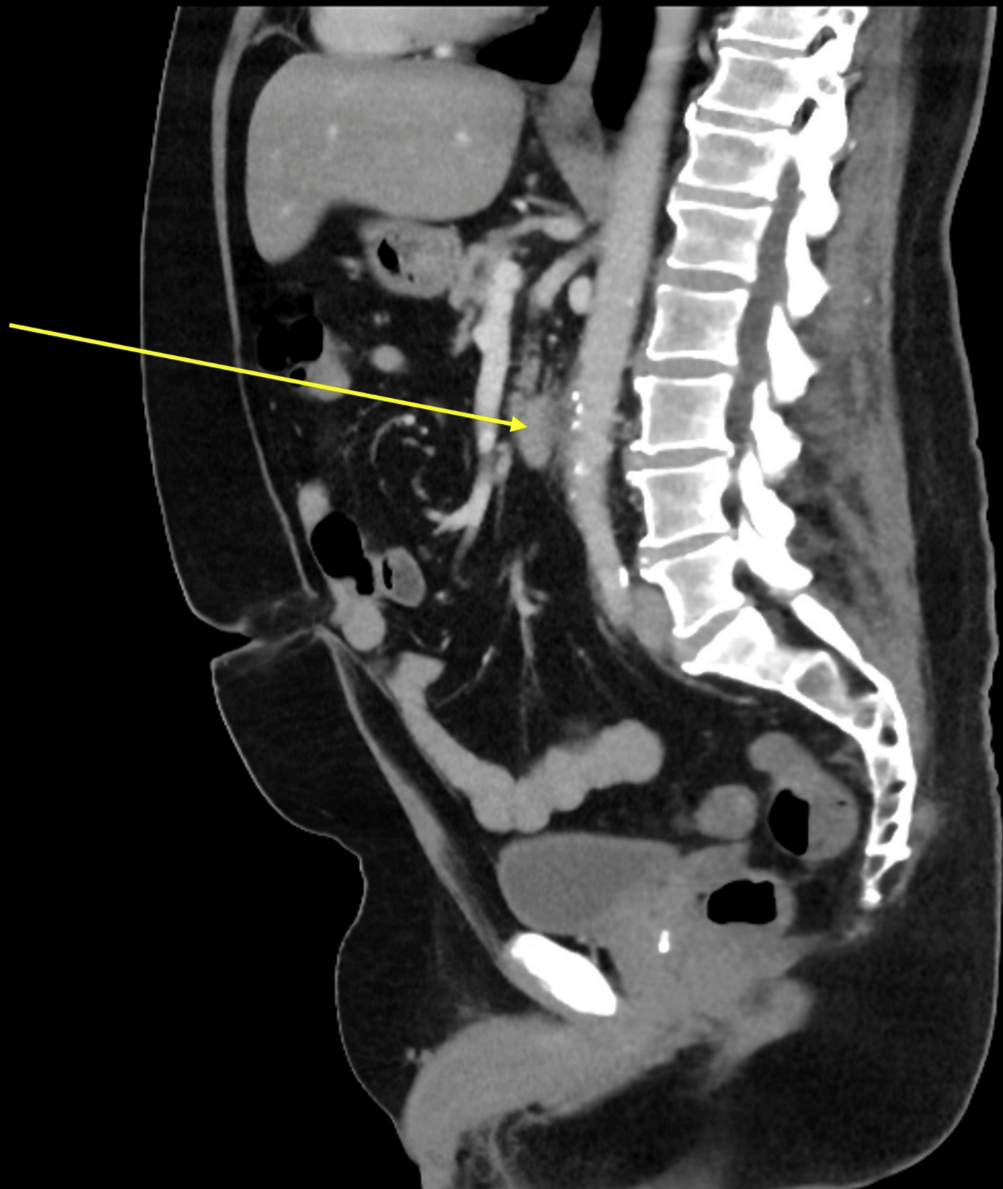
Sagittal contrast-enhanced CT of the abdomen and pelvis showing fusiform bulging of the mid-abdominal aorta with surrounding fat stranding and calcific changes, consistent with early aortitis or atherosclerotic dilatation.
